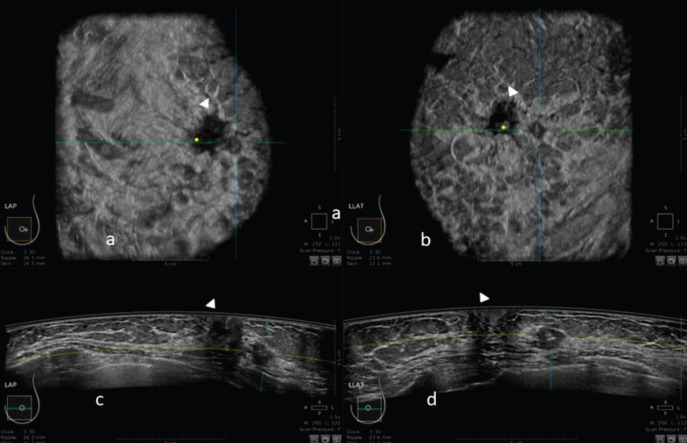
3D ultrasound image at the dedicated workstation of a 82-year-old patient with biopsy proven ductal infiltrating carcinoma. a and b: reconstructed coronal plane; the lesion is marked as a reference point. Arrowhead shows the nipple. c and d: axial plane: the lesion is marked as a reference point. Arrowhead shows the nipple.
